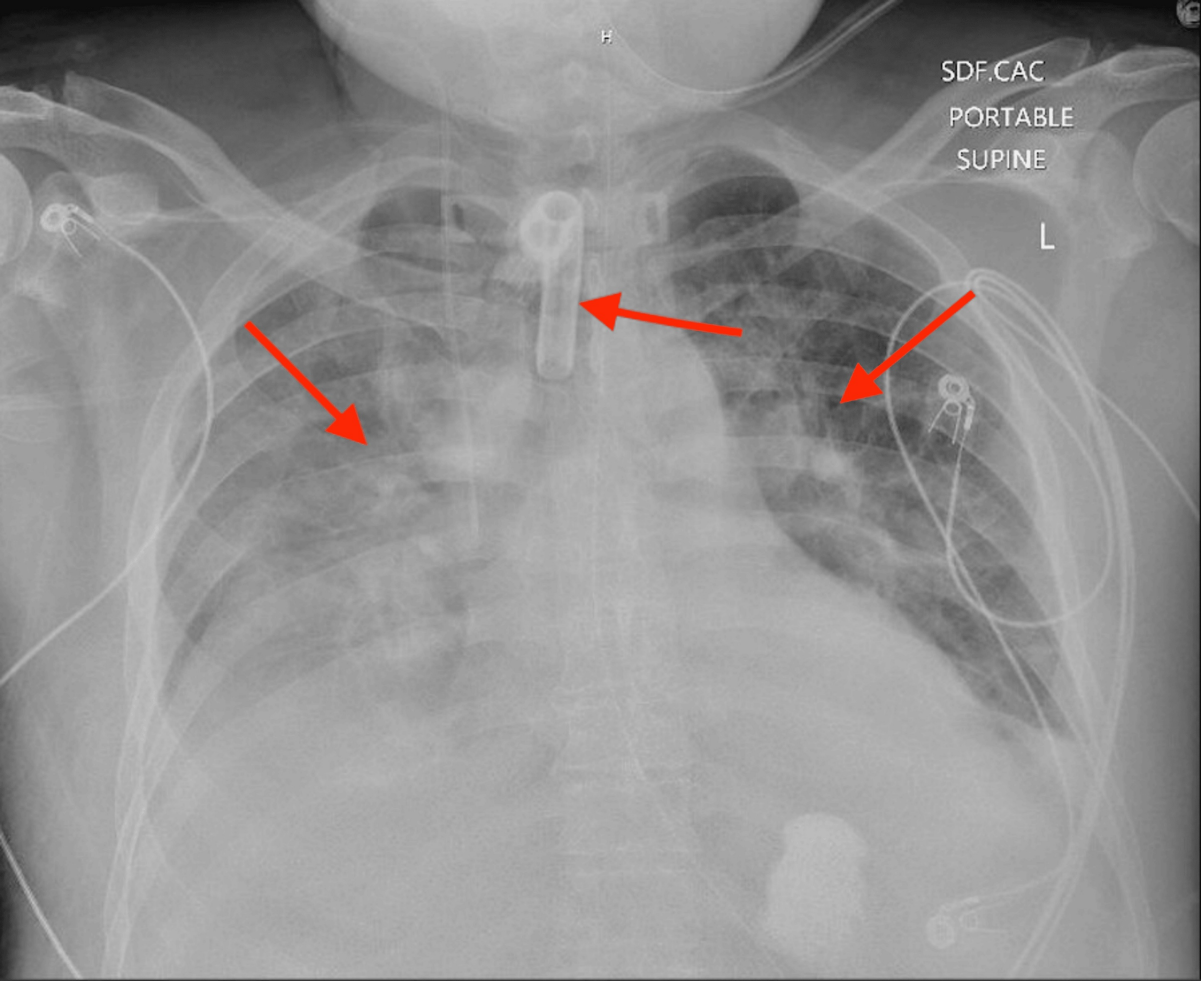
Portable chest radiograph demonstrating a cuffed tracheostomy tube (middle arrow) with bilateral diffuse pulmonary infiltrates (left and right arrows), consistent with acute hypoxic respiratory failure in the setting of influenza A and septic shock.
